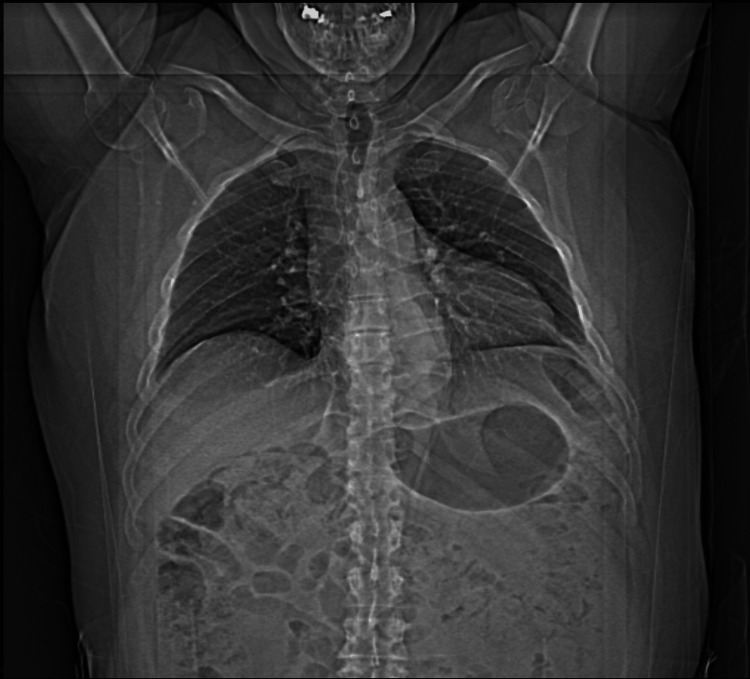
Postoperative chest X-ray after hernia repair
